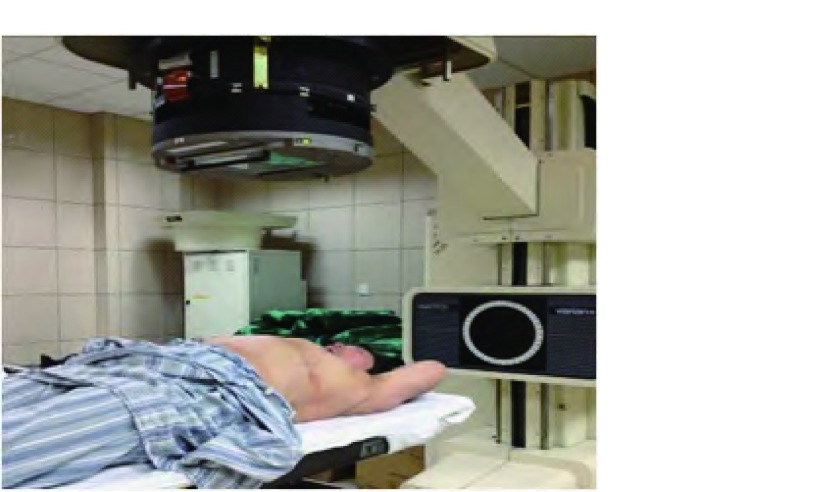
模拟机上复制CT扫描时的体位 Re-relization the position of CT scan on analog machine in the red squrel.
